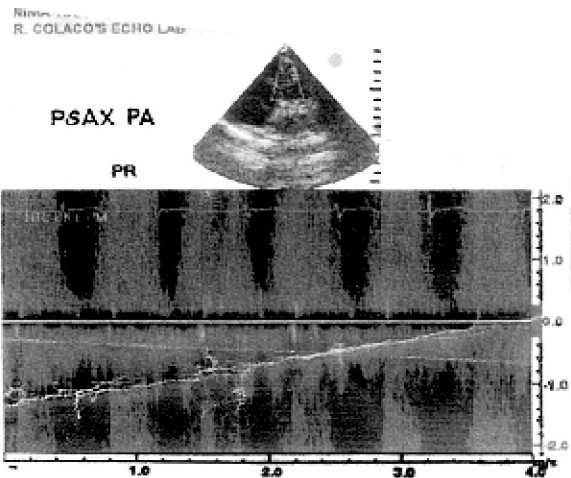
Transthoracic echocardiogram in parasternal short axis (PSAX) view shows a continuous- wave Doppler flow imaging demonstrate a continuous left to right shunt with a high-velocity systolic and diastolic flow signal. PA=Pulmonary Artery, PR=Pulmonary Regurgitation.
